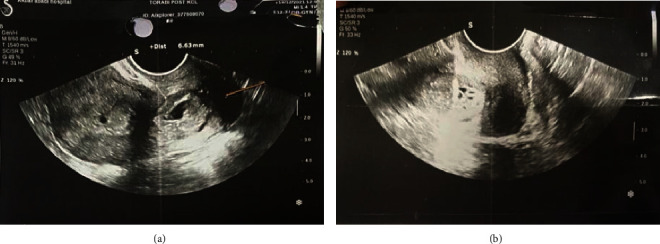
The obstetric ultrasonography shows the pregnancy sac and the yolk sac with the embryonic pole located near the internal os.
